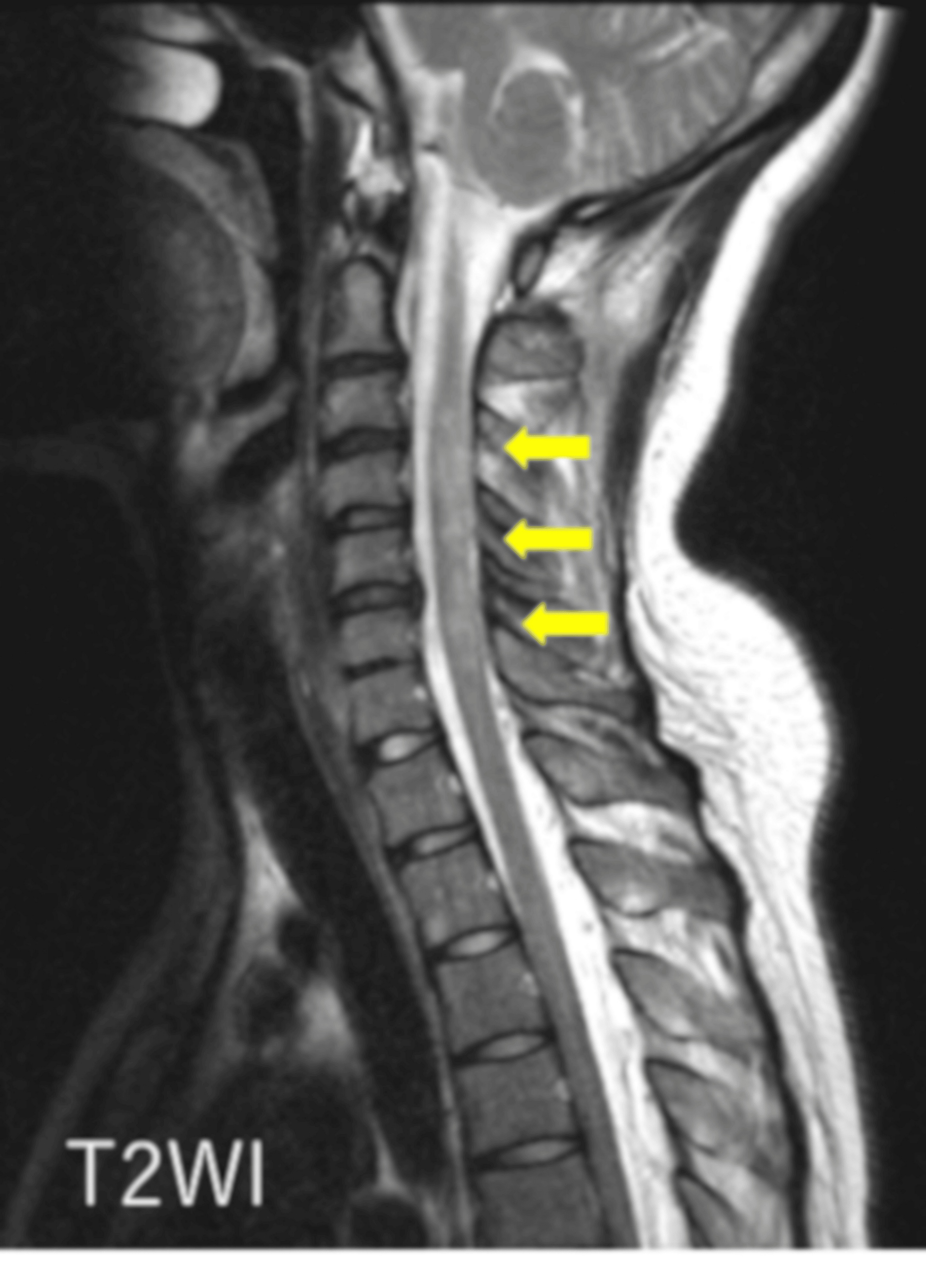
Sagittal non-contrast MRI of the cervical spine Sagittal T2-weighted cervical spine MRI demonstrating a hyperintense lesion extending from C2 to C6 (yellow arrows).
